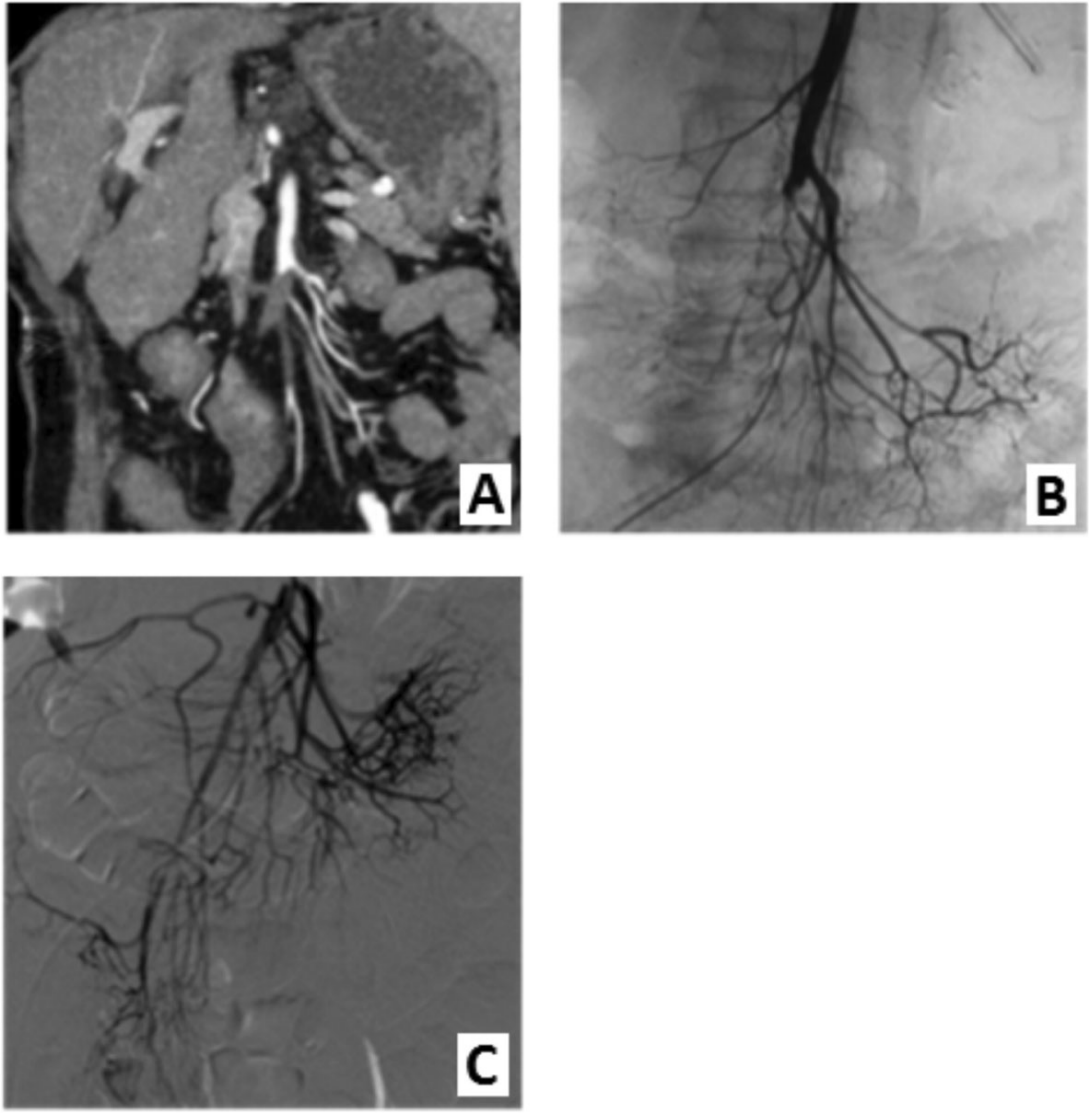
SMA embolism in proximal side branches. a CT image, coronal view, arterial phase. b An-giography showing disrupted blood supply particularly to the ileocolic artery and branches. c Restored blood supply in affected segments after thrombosuction, thrombolysis and PTA
